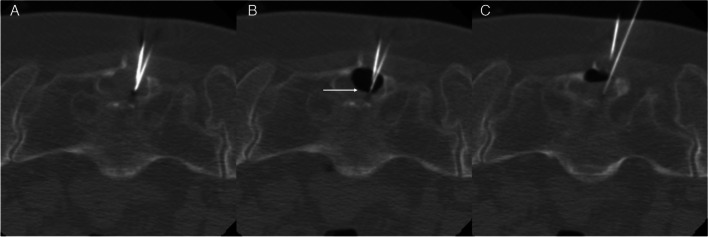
Two-needle technique for therapeutic aspiration and fibrin sealant injection of a symptomatic right S3 Tarlov cyst. A Two 22G spinal needles were advanced into the cyst under CT fluoroscopic guidance with one needle placed deep and the other more superficially. The stylets were then removed. B Aspiration from the needle placed in the deep aspect of the cyst was performed which produced a stable air-fluid level within the cyst (arrow). C Fibrin sealant (Tisseel VH; Baxter Healthcare, Westlake Village, California) was then injected into the cyst, with the volume injected equating to 80% of the aspirate volume
